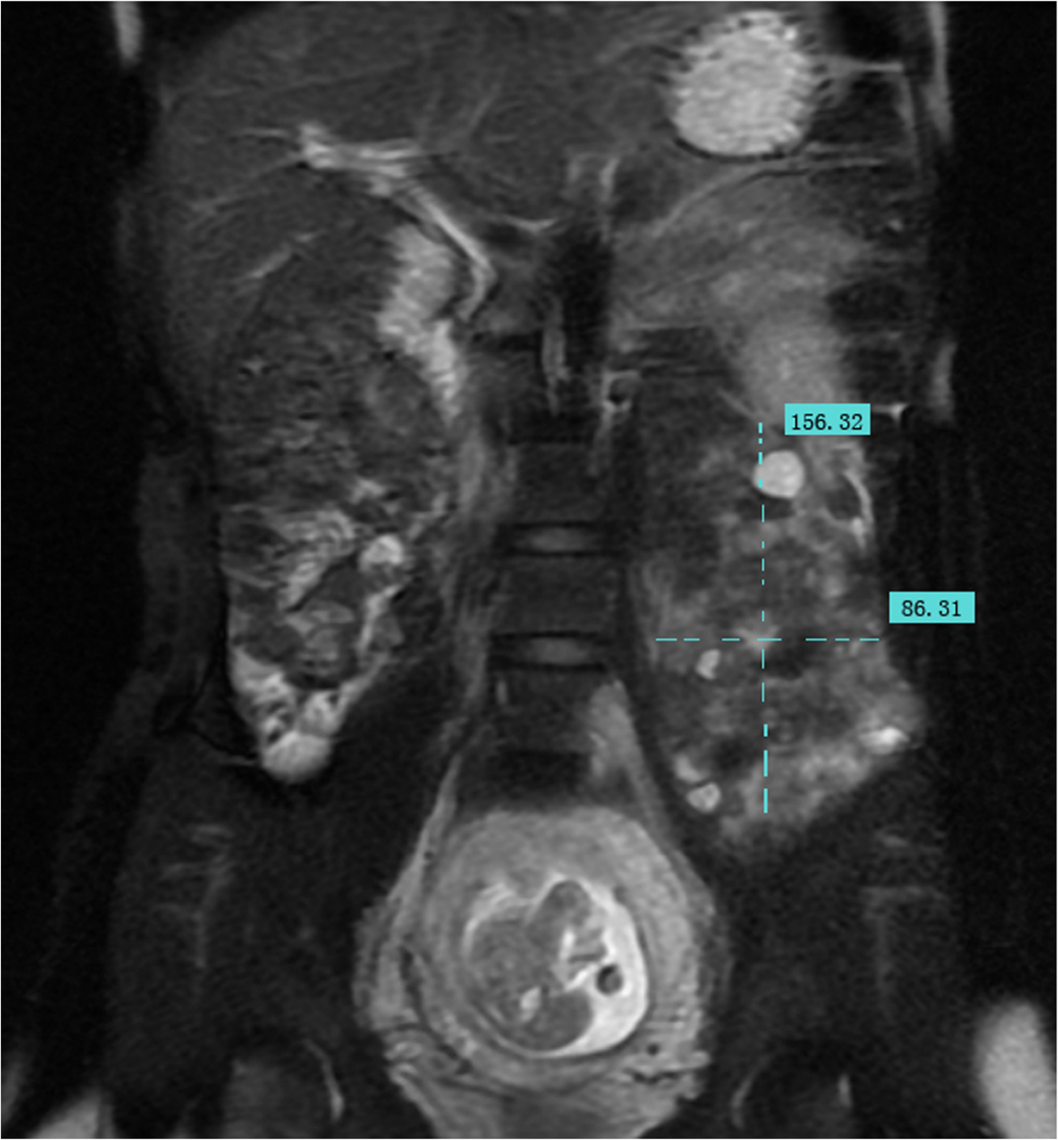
MRI (Coronal T2-FRFSE Fat SAT). The extend of the tumor and relation with adjacent organs (1).
